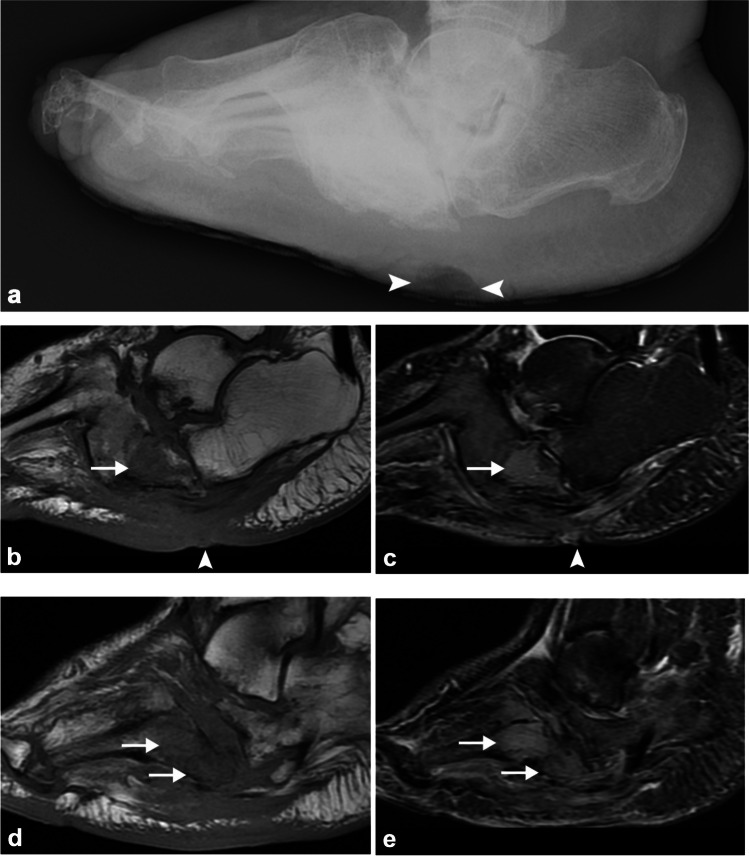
Fifty-six-year-old diabetic man with left foot ulcer and soft tissue swelling. Lateral radiograph (a) demonstrates disorganization, relative increased density, dislocations, and rocker-bottom deformity of the midfoot, compatible with neuropathic arthropathy (Charcot foot) with a plantar soft tissue ulcer (*arrowhead*). Sagittal T1 (b) and STIR (c) MR images at the level of the cuboid and sagittal T1 (d) and STIR (e) MR images at the level of the fourth and fifth metatarsal bases demonstrate bone marrow replacement with increased STIR and decreased T1 signal intensity in the cuboid and proximal fourth and fifth metatarsal bases (*arrows*) compatible with superimposed osteomyelitis subjacent to the ulcer
